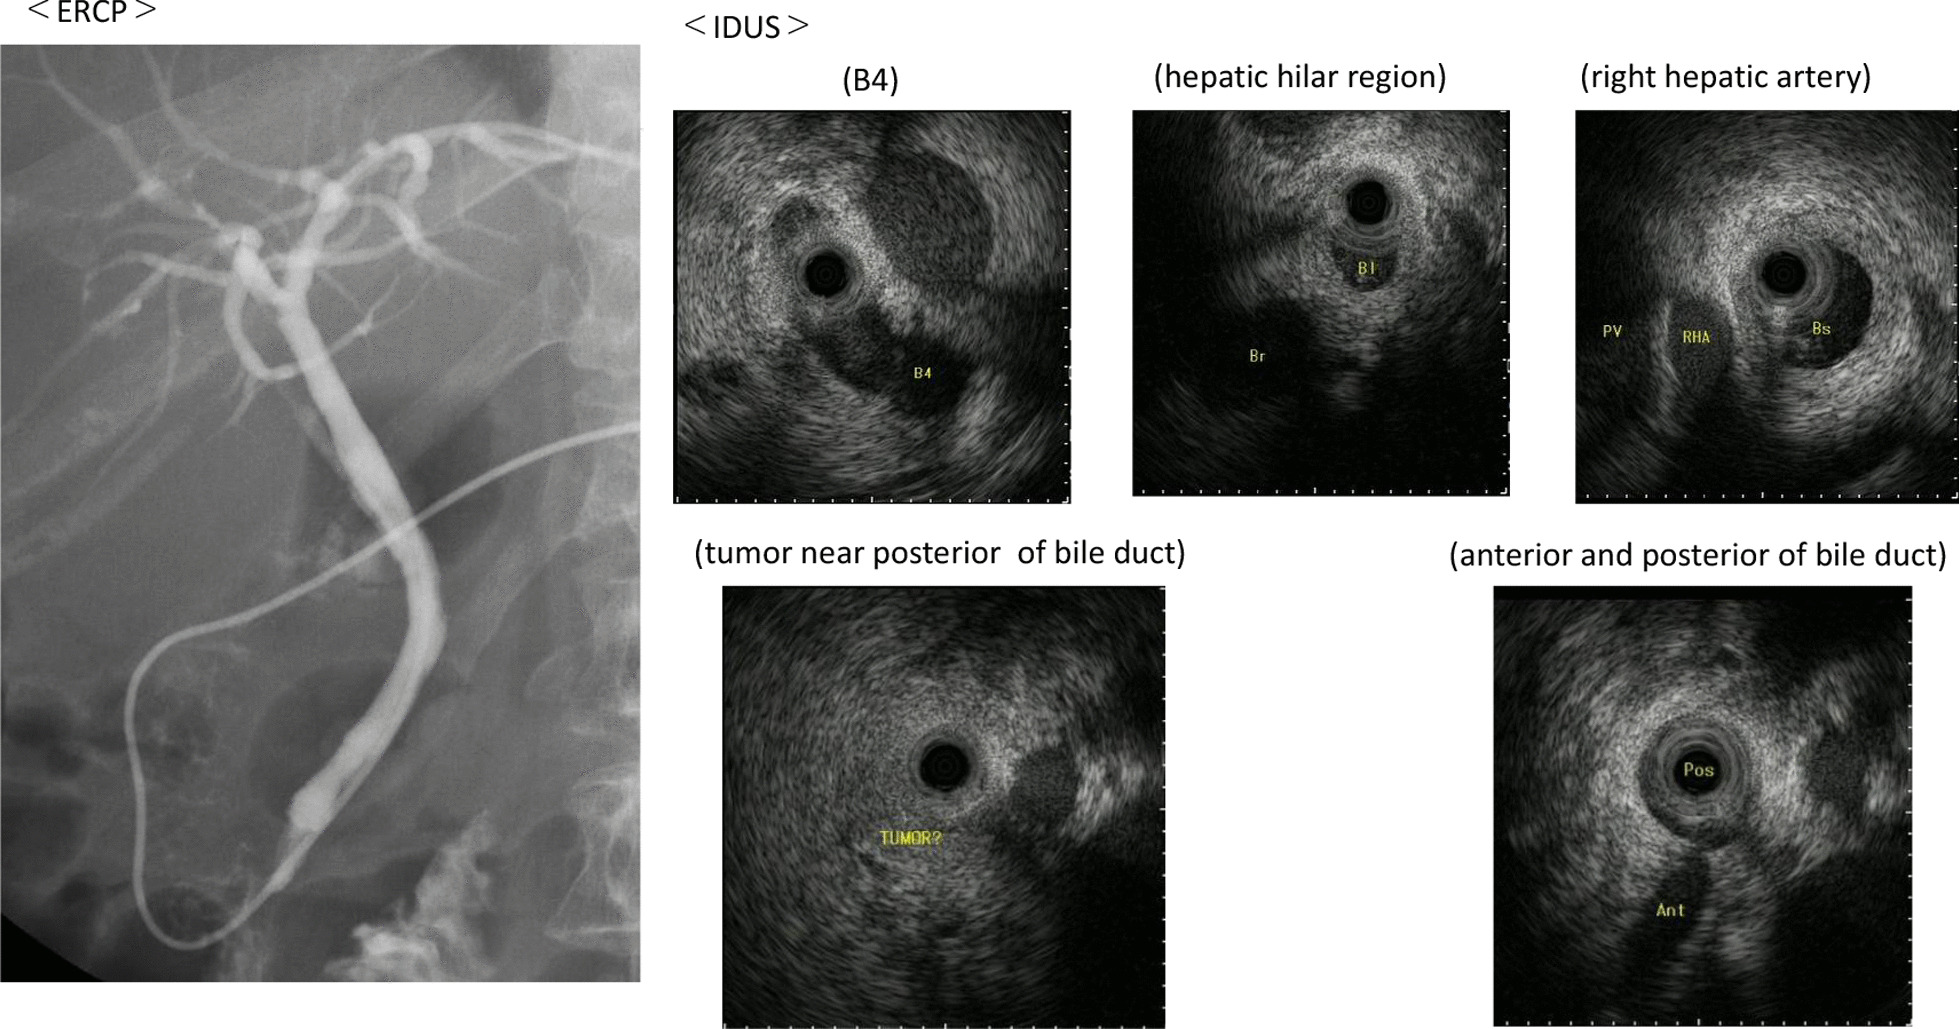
Intraductal ultrasonography revealed localized wall thickening in the extra-pancreatic ducts starting at the branch of the left and right hepatic ducts. Biopsy of bile duct mucosa found no malignant cells in the branch of the left and right hepatic ducts, the left hepatic duct, or the intrapancreatic ducts.
